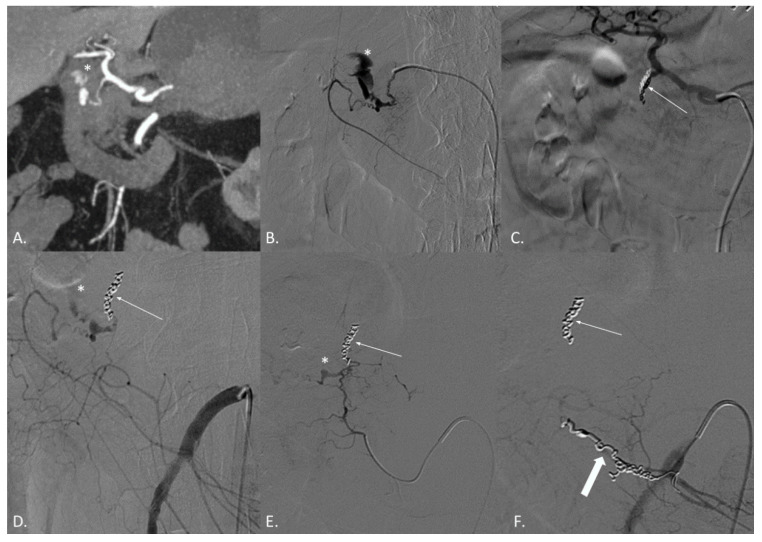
(A–F) CT angiography (CTA) and digital subtraction angiography (DSA) of a 74-year-old male patient suffering from active bleeding of a duodenal peptic ulcer successfully treated with coil embolization both from the celiac trunk and the superior mesenteric artery (SMA)—“sandwich technique”. (A) CTA demonstrates active endoluminal bleeding (*) from the gastroduodenal artery (GDA). (B) DSA obtained with catheterization of the GDA confirms CTA findings. (C) DSA obtained with catheterization of the celiac trunk after coils and gelfoam embolization of the GDA (thin arrow). (D) DSA obtained with catheterization of the SMA demonstrating residual active bleeding (*) from the inferior pancreaticoduodenal arteries (iPDA). (E) DSA obtained with catheterization of the iPDA confirms the active bleeding (*). (F) DSA obtained with catheterization of the SMA after coil embolization of the iPDA (thick arrow). No active bleeding evidence.
